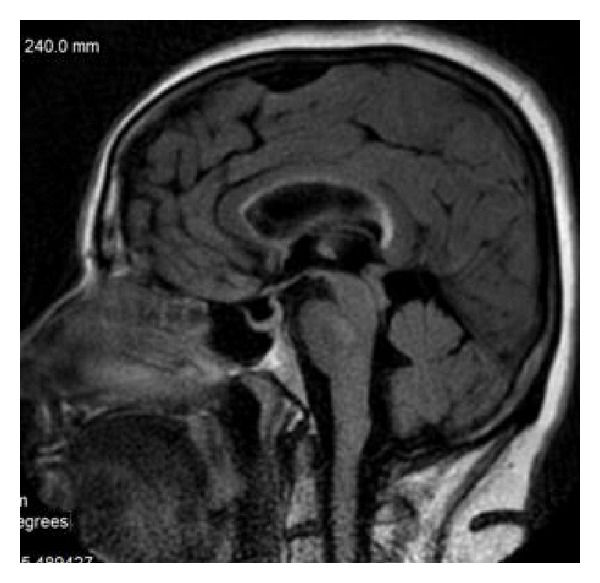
FLAIR, sagittal plane. Hyperintense band in the ventral part of CC in a 58-year-old woman with uncontrolled hypertension.
